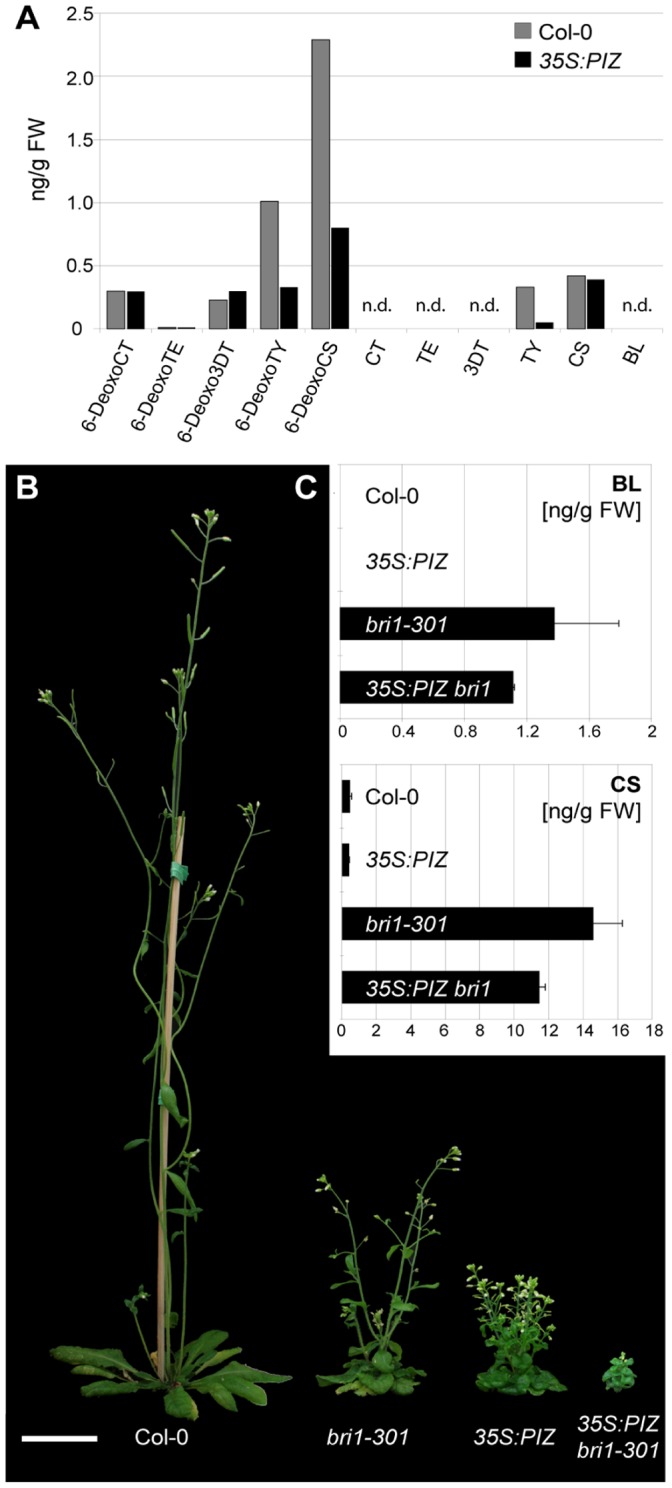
Endogenous BR levels in *35S:PIZ* and *35S:PIZ bri1-301* seedlings. (A) Free BR levels in aerial tissues of 4-week-old *35S:PIZ* seedlings quantified by GC-MS are shown in ng/g fresh weight (FW). 6-deoxo-cathasterone (6-deoxoCT), 6-deoxo-teasterone (6-deoxoTE), 3-dehydro-6-deoxoteasterone (6-deoxoDT), 6-deoxo-typhasterol (6-deoxo-TY), 6-deoxo-castasterone (6-deoxoCS), cathasterone (CT), teasterone (TE), 3-dehydro -teasterone (3DT), typhasterole (TY), castasterone (CS), brassinolide (BL). n.d., not detected. (B) Growth phenotypes of 17-day-old Col-0, *bri1-301, 35S:PIZ* and *bri1-301 35S:PIZ* (from left to right). Scale bar represents 3 cm. (C) Free BL and CS levels from aerial tissues of 17-day-old plants are analysed by LC-ESI-MS/MS. Mean values of three biological replicates are shown, relative to fresh plant weight (FW), with SD.
